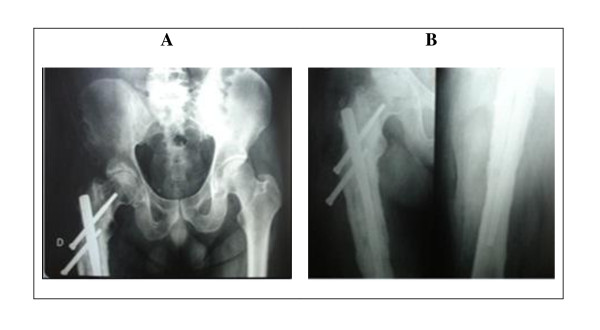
Case 1 - Radiographic images of the pelvis in anteroposterior view (A), and the hip joint in anteroposterior and lateral view (B), showing the subtrochanteric fracture of the right femur consolidated in varus angulation. The reduction in varus and the intense periosteal reaction caused by infection can be observed. The entry point of the nail is excessively lateral, and the interlocking screws are short and incorrectly positioned culminating in the lateral migration of the inferior screw (Z-effect).
